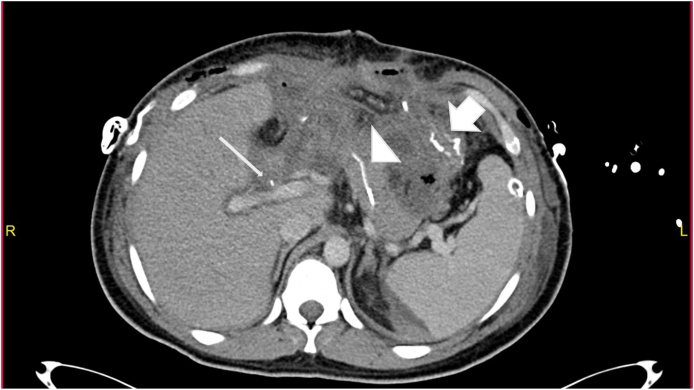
Axial enhanced CT scan of the abdomen post whipple procedure showing; A: gastrojejunostomy (Bold arrow), B: pancreaticojejunostomy(arrowhead), C: Choledochojejunostomy (thin arrow).
